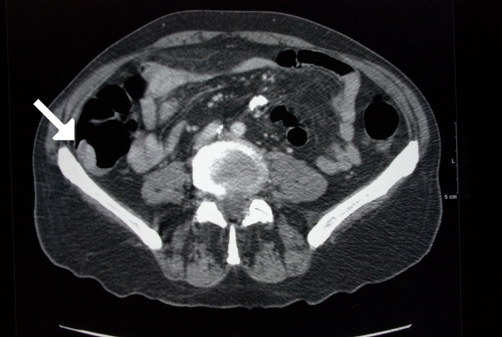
Abdominal CT showing a polyp in the ascending colon (arrow) in an 86 years-old male patient.
